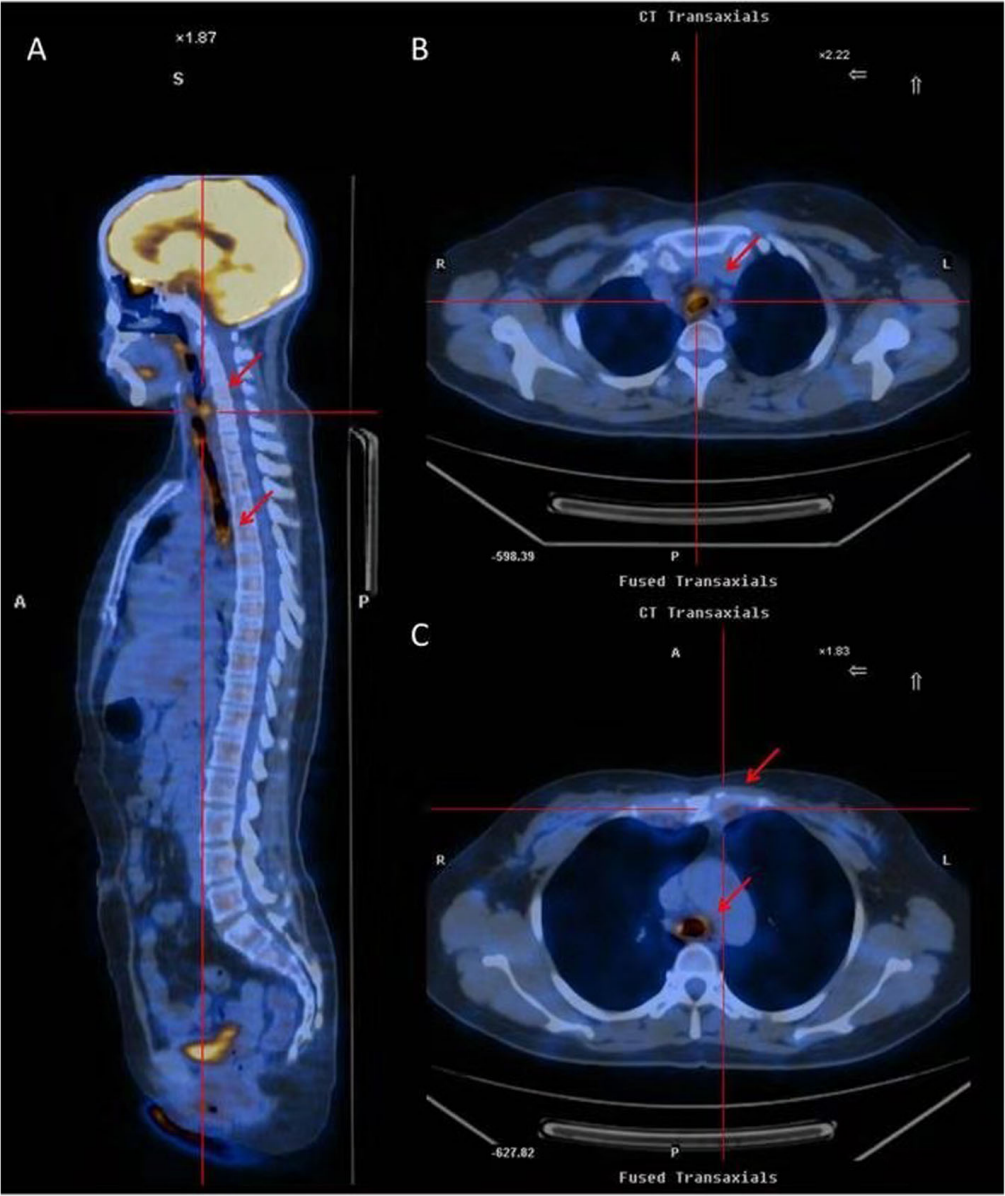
A 44-year-old woman with RP showed multicartilage involvement. a A whole-body view showed multiple foci of pathological tracer uptake in cartilages (vocal cord, SUV_max_ 5.8; tracheal/bronchial cartilage, SUV_max_ 5.8; red arrowhead). b The SUV_max_ of the tracheal cartilage was approximately 5.8 (red arrowhead). c Abnormal uptake was detected in the costicartilage and tracheal cartilage (red arrowhead)
